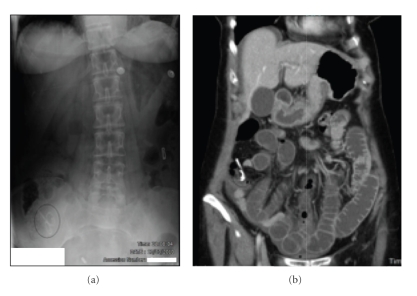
(a) Abdominal X-ray. No free intra-abdominal air. Note IUCD in RIF. (b) CT scan of the abdomen.
